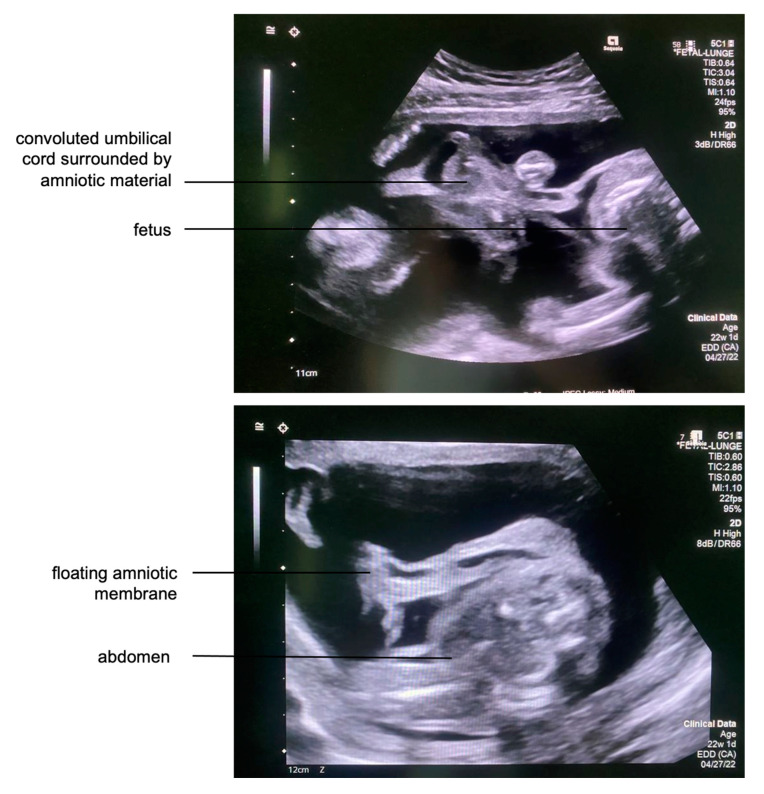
Top*:* Transabdominal fetal ultrasound showing multiple strands of membranous material encircling and partially constricting the umbilical cord, indicative of a life-threatening state for the fetus. Bottom*:* Due to subtotal chorioamniotic membrane separation, the subtotally disrupted amniotic membrane floated freely next to the fetus.
